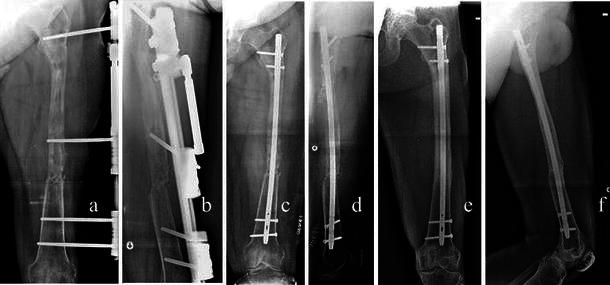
Case 2: a, b preoperative X-ray, c, d postoperative checkup, e, f follow-up 5 years later
